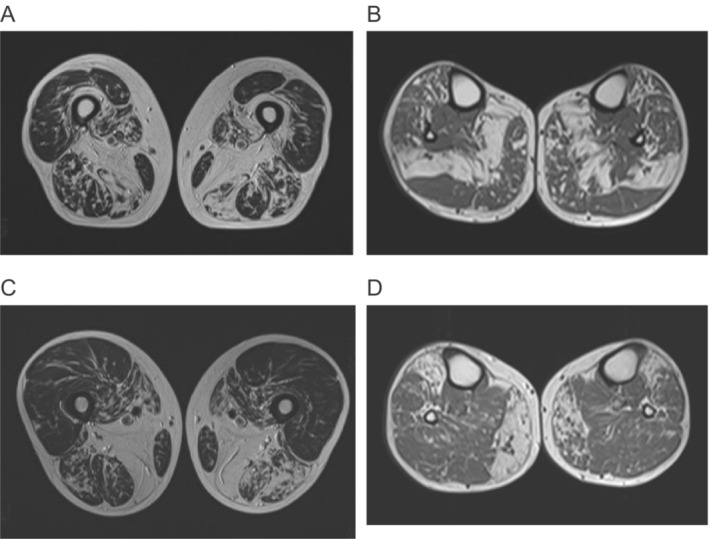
Magnetic resonance imaging (MRI) of F1.III:12 (A‐B) and F1.IV:4 (C‐D). F1.III:2, at age 48 years, showed severe fatty involvement of vastus intermedius and adductor magnus on the thigh with milder fatty involvement in all hamstrings, adductor longus, and vastus medialis (A). The soleus is severely involved with milder changes in tibialis anterior and medial gastrocnemius (B). In the thigh of F1.IV:4 adductor magnus is replaced with milder fatty involvement of hamstrings, vastus medialis, and sartorius (C). On the lower legs, the medial gastrocnemius is asymmetrically, while the tibialis anterior is more symmetrically involved (D).
